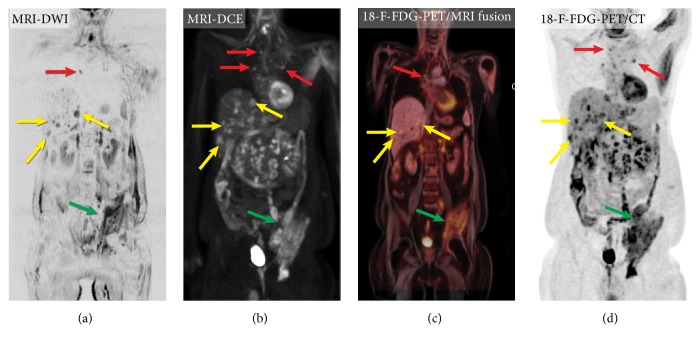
Imaging of metastatic medullary thyroid carcinoma using different radiological techniques: (a) whole-body magnetic resonance imaging, diffusion-weighted sequence (DWI), (b) whole-body contrast-enhanced magnetic resonance imaging, T1 weighted images (3D rendering DCE); (c) positron emission tomography/magnetic resonance imaging (18F-FDG-PET/MR imaging fusion technique); (d) positron emission tomography/computed tomography with 18F-FDG (18F-FDG PET/CT). Please note the excellent visualization of the multiple liver metastases (yellow arrow), mediastinal metastases (red arrow), and a large left iliac bone metastasis (green arrows) on DWI and DCE, comparable with 18F-FDG PET/CT, although they interrogate completely different biophysical tissue properties. Note that the images are not quite coregistered (arms up during PET acquisition and with arms down during MR imaging).
